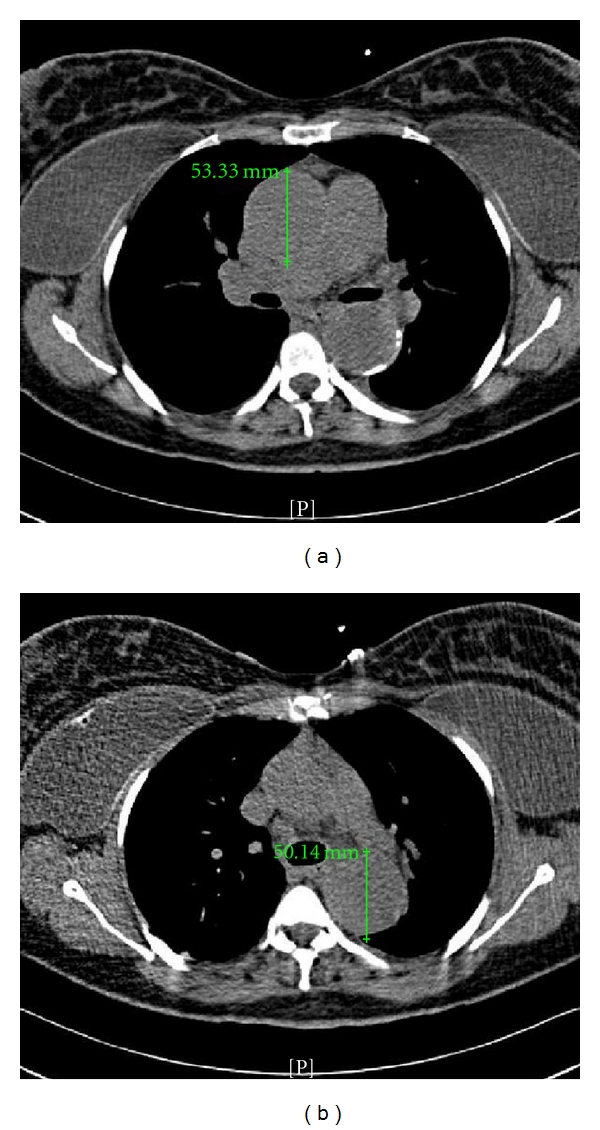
Radiographic changes with aortic dilatation. (a) Shows the ascending aorta measuring 5.3 cm in diameter. (b) Shows the descending aorta measuring 5.0 cm in diameter.
